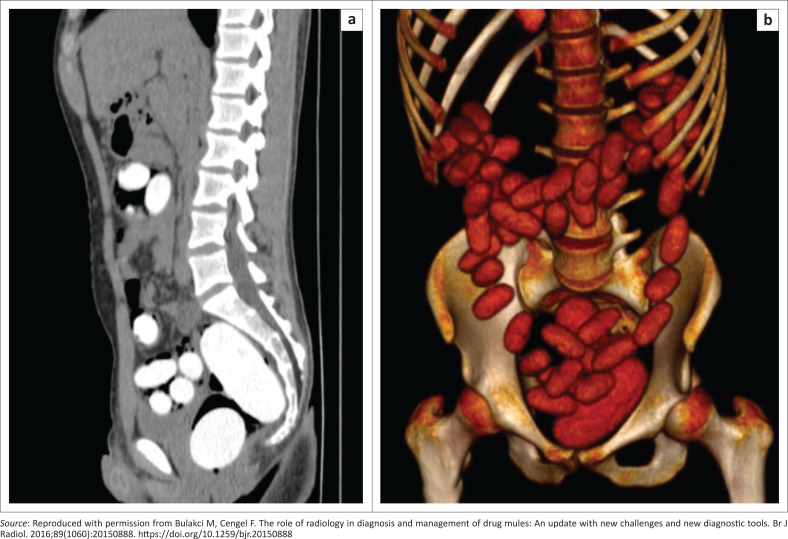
A 25-year-old male body packer and body pusher. (a) Sagittal computed tomography imaging of the intestinal loop lumens without contrast material revealed multiple hyperdense drug-filled capsules along with two bigger sized packages in the lumen of the rectum. (b) Three-dimensional volume-rendering technique images distinguish the capsules more easily. A total amount of 1093 g of 60 cocaine capsules were obtained from this case.
